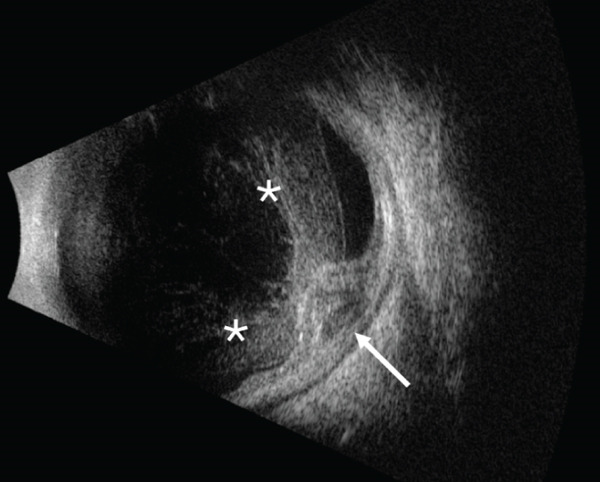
The B‐scan ocular ultrasound imaging showed hyper‐reflective material in vitreous chamber (asterisks) and subretinal mass with high nonhomogeneous echogenicity projecting into the vitreous (arrow).
